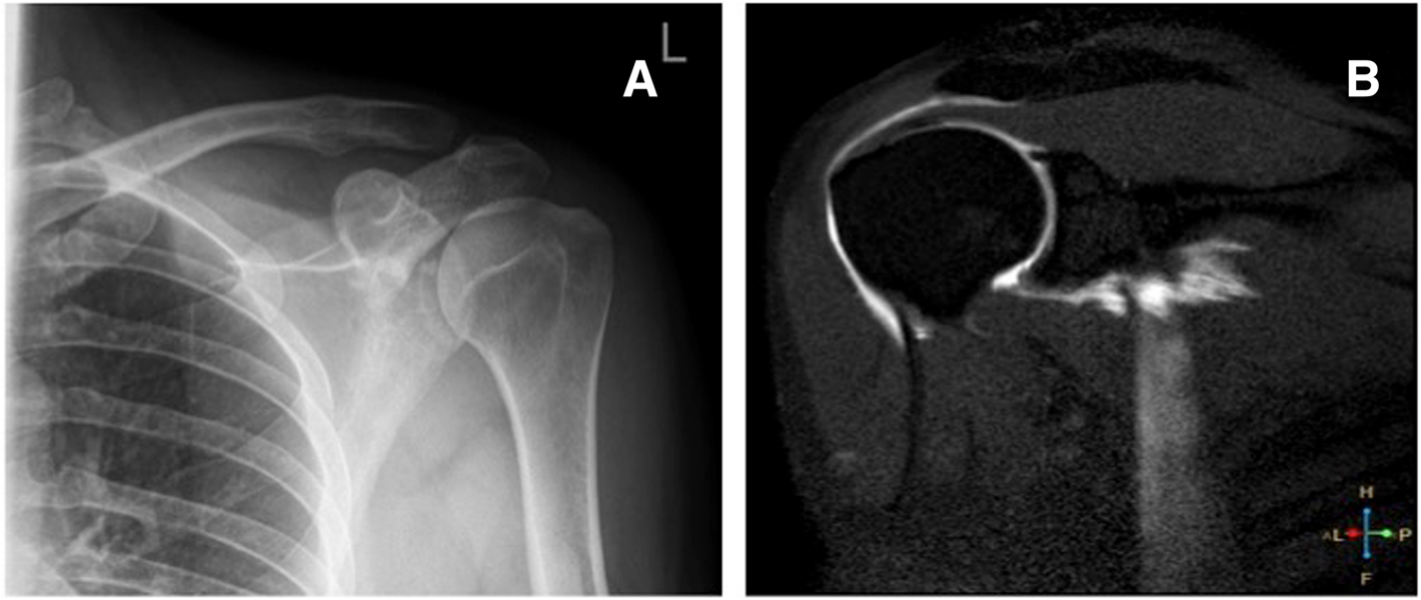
a Plain radiograph in coronal plane left shoulder. No acute abnormality reported. Incidental calcific deposit noted. b MR arthrogram coronal plane of same patient showing tear of subscapularis tendon and leakage of contrast agent
